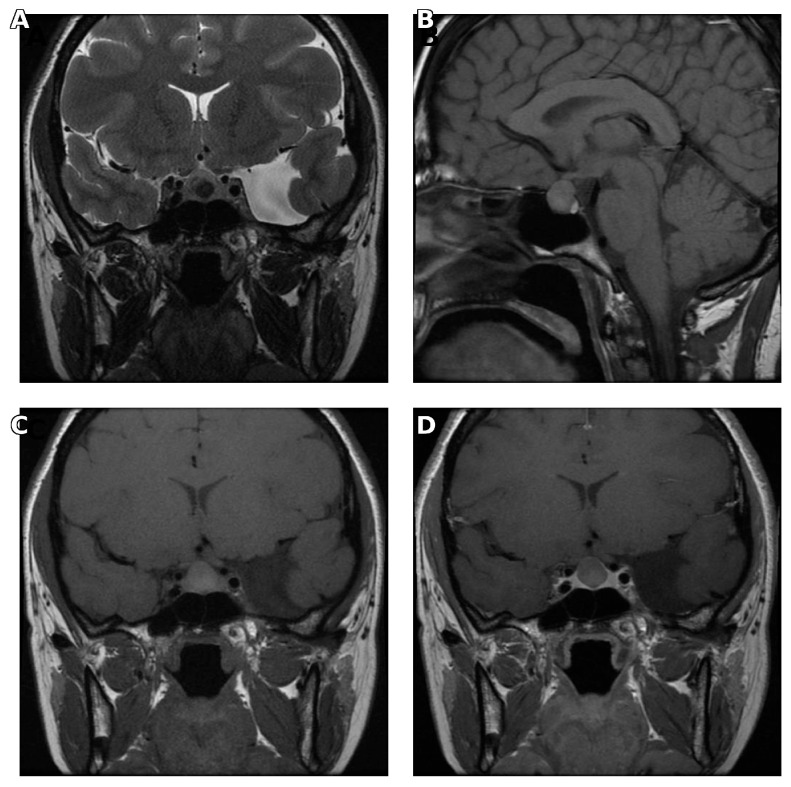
(A) Coronal T2-weighted image demonstrating an intracystic hypointense nodule. (B) Contrast-enhanced sagittal T1-weighted image demonstrating a midline-located Rathke’s cleft cyst. (C) Coronal T1-weighted image demonstrating the intracystic nodule. (D) Contrast-enhanced coronal T1-weighted image demonstrating no enhancement of the intracystic nodule.
